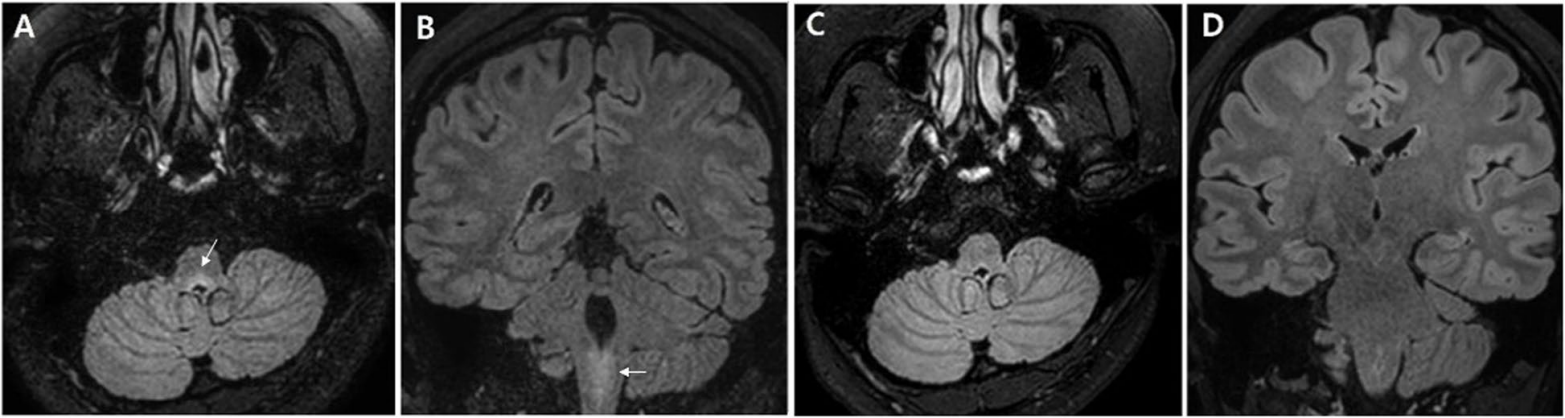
A, B Fluid attenuated inversion recovery (FLAIR) images showing a focal hyperintense lesion in the medulla adjacent to the foramen of Magendie (arrows). C, D Follow-up MRI taken 22 weeks later showing a marked improvement
